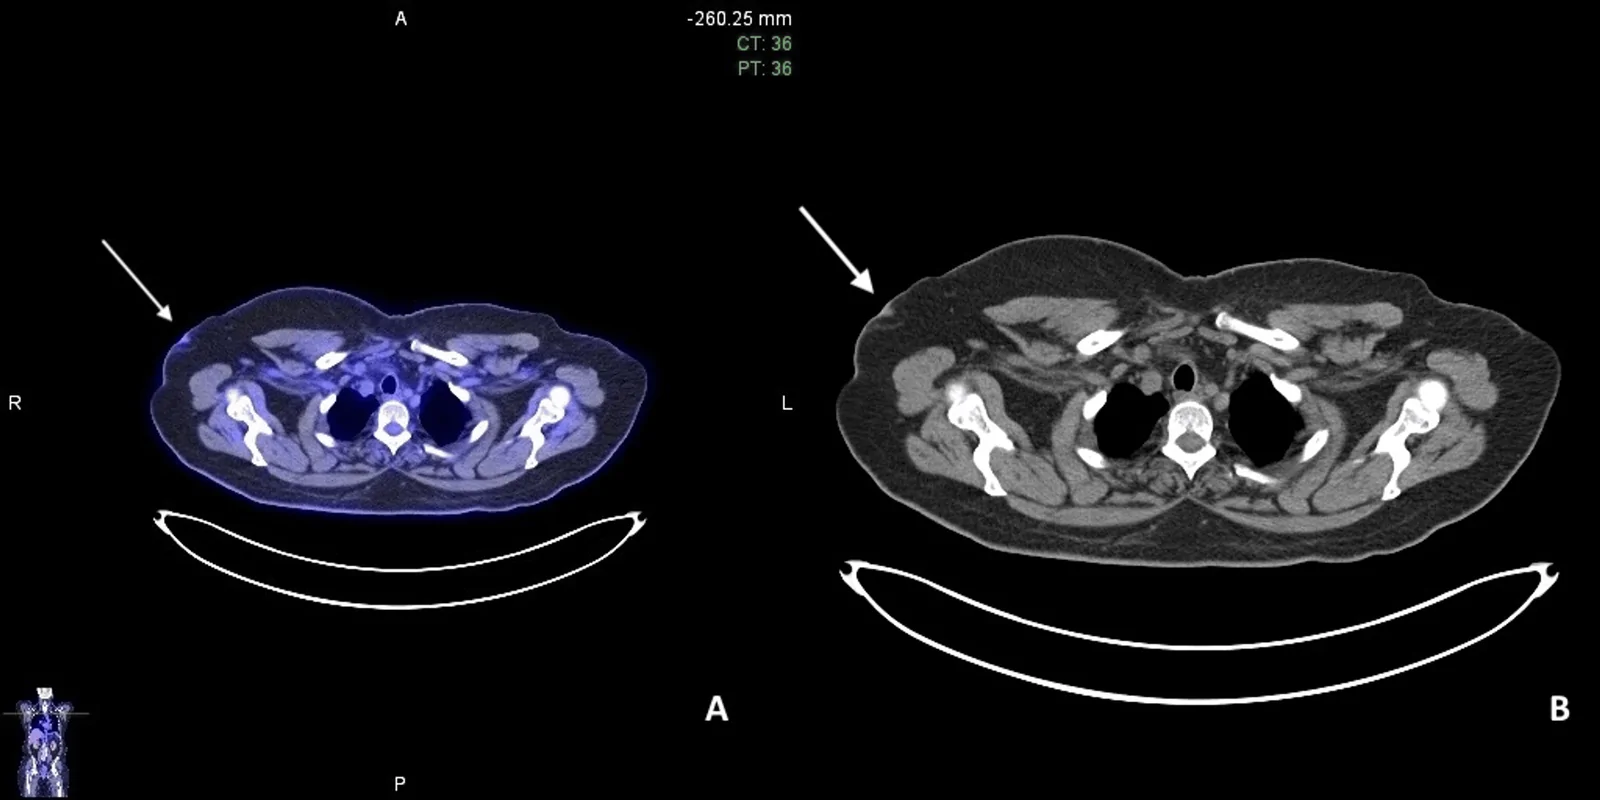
PET-CT shows only mild metabolic activity within the right axilla lesion (A and B, maximum standardized uptake value (SUVmax) 2.7), with no evidence of synchronous lesion within either orthotopic breast, regional nodal involvement or distant metastatic disease.
